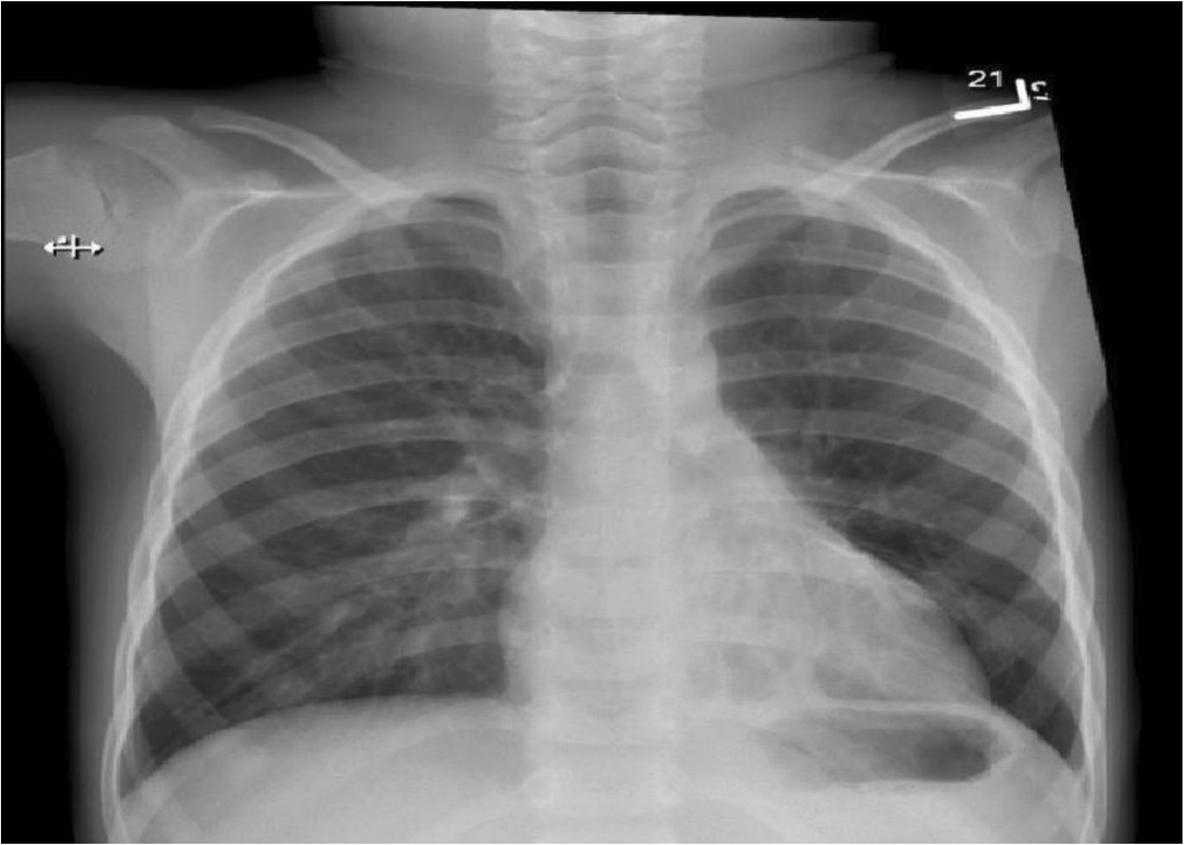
Chest X-Ray 6 months after Bronchoscopy showing a significant varicose bronchiectasis in the left lower lobe with interval improvement of the consolidation in the same lobe
